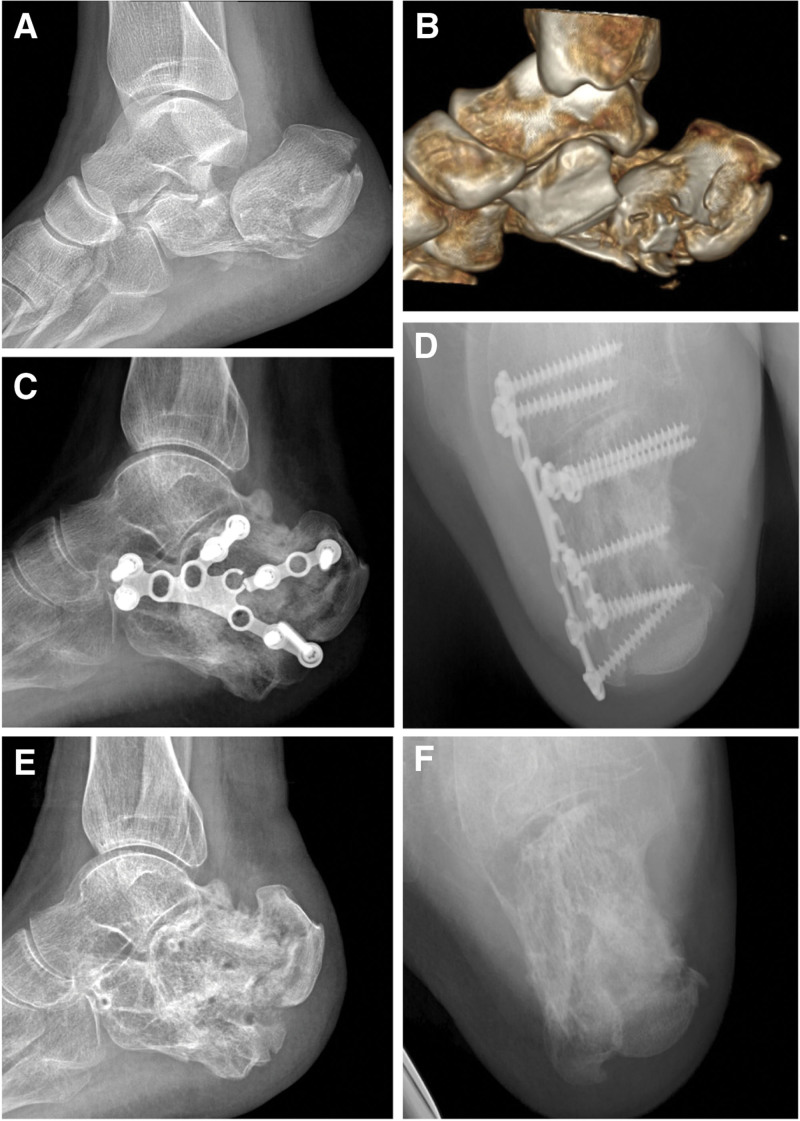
A 31-year-old male patient fell from a height of 3 meters. (A and B) A preoperative lateral radiograph and computed tomography (CT) image showing right calcaneus fracture (Sanders type ⅢAC, Tough type) with unnormal Böhler angle (–33°), Gissane angle (116°), height (82.4 mm), and length (83.4 mm). 3-ram straight plate fixation via the L-shaped approach was used. (C and D) At the last follow-up, the lateral and axial radiographs showed the Böhler angle (12°), Gissane angle (117°), height (82.5 mm), length (83.6 mm), and width (41.9 mm) are not well restored. (C, E and F) The patient experienced plate breakage and calcaneal malunion, and the AOFAS score was 49. AOFAS = American Orthopedic Foot and Ankle Society.
